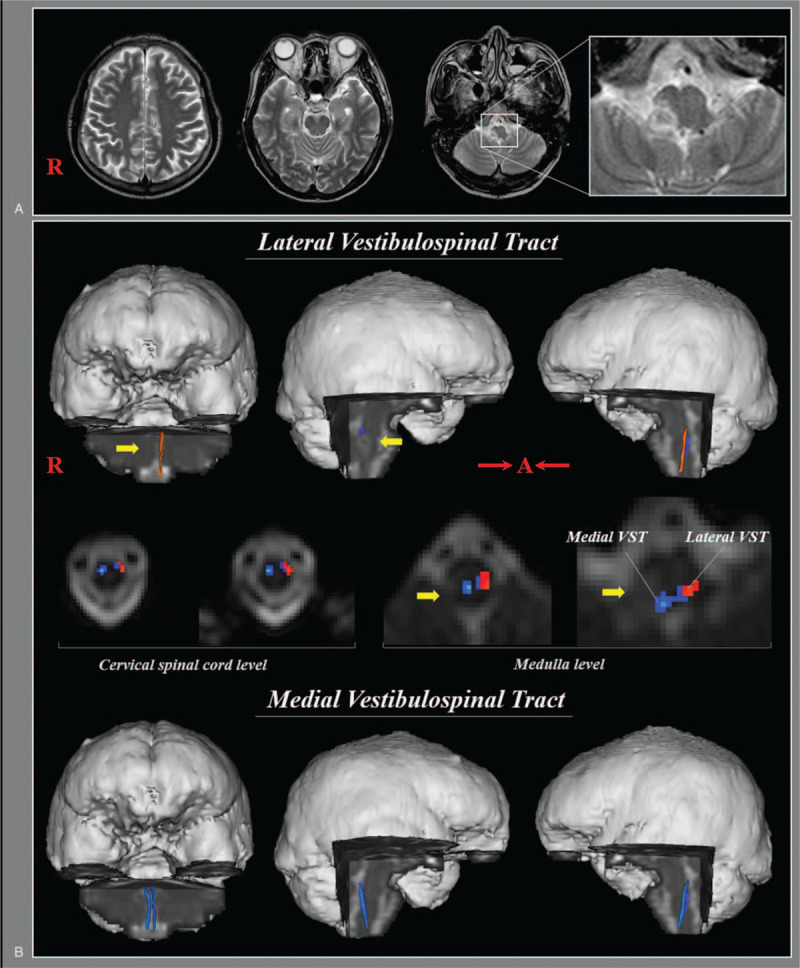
(A) Brain MR images at 2 weeks after onset show an infarction in the right dorsolateral medulla. (B) Results of diffusion tensor tractography (DTT). DTTs for both the medial vestibulospinal tract (VST) and the left lateral VST originate from the pontine vestibular nuclei and terminate at upper cervical cord. By contrast, DTT for the right lateral VST is not reconstructed between the lateral vestibular nuclei in pons and the upper cervical cord.
